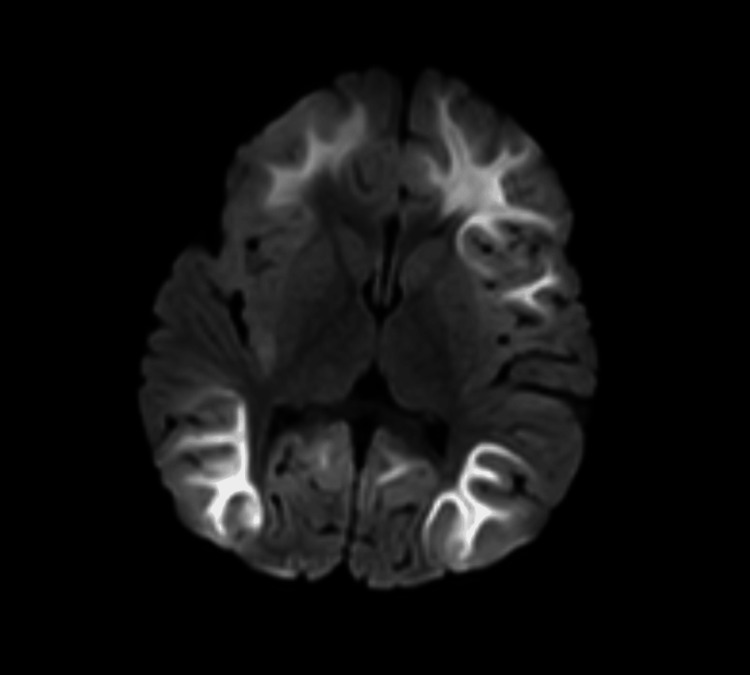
Representative brain MRI — diffusion-weighted imaging (DWI) demonstrating ALERD in case 6 (patient 6: 17-month-old male with enteroviral encephalitis). Axial DWI brain MRI from Case 6 (17-month-old male) demonstrating extensive bilateral symmetric areas of bright diffusion restriction (high signal on DWI) involving the subcortical and periventricular white matter of the bilateral posterior frontal and parietal lobes, with additional involvement of the occipital white matter. These findings are consistent with acute leukoencephalopathy with restricted diffusion (ALERD), a rare but distinct pattern of brain injury associated with severe enteroviral encephalitis. In the appropriate clinical context, acute viral illness, CSF PCR-confirmed enterovirus, and rapid neurological deterioration, these bilateral symmetric white matter diffusion abnormalities are characteristic of ALERD and carry prognostic significance for adverse neurological outcomes. Sequence: DWI b=1000. The patient was 17 months of age at the time of imaging.
